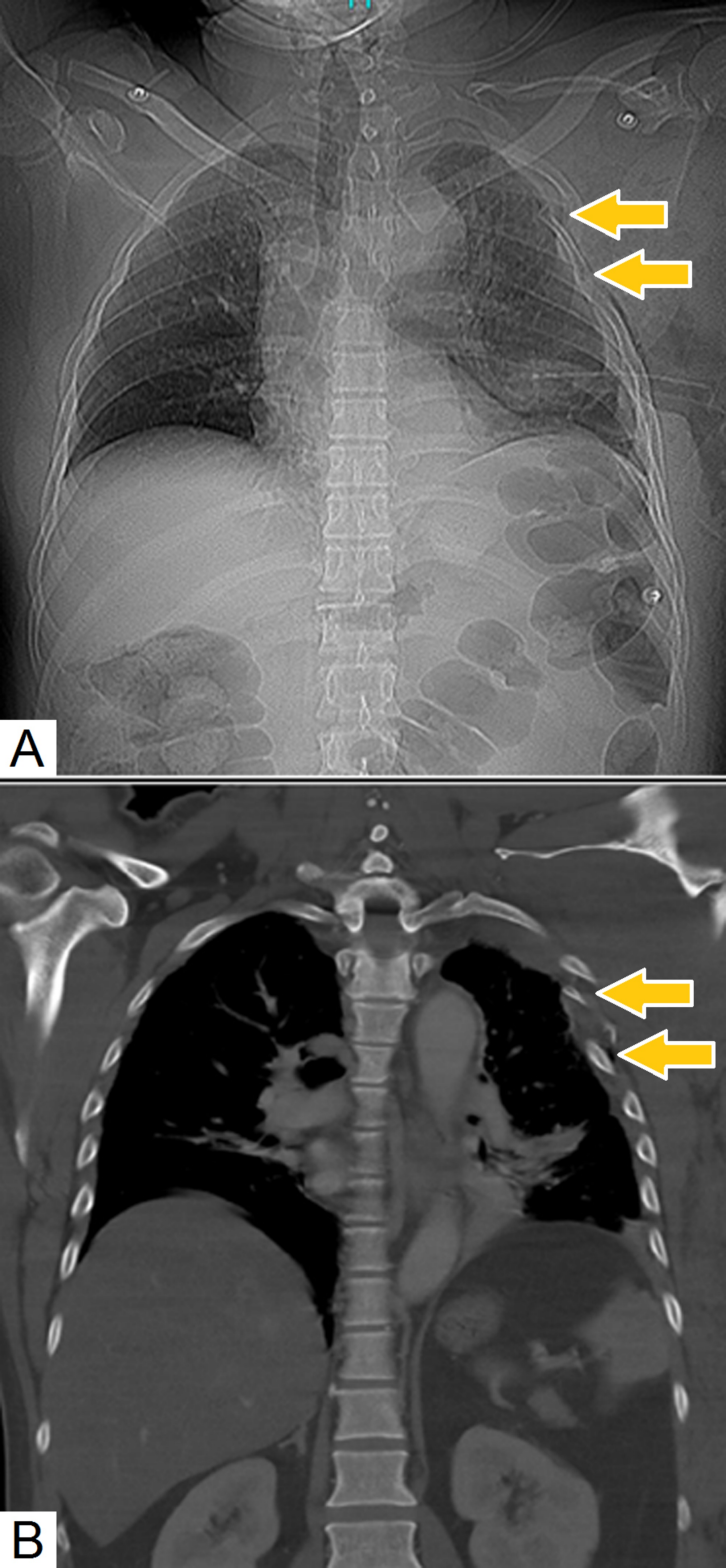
Comparison of scout film and thoracic computed tomography (A) Scout film demonstrating multiple left-sided rib fractures (arrows). (B) Coronal chest computed tomography (CT) images confirm the presence of multiple left-sided rib fractures (arrows).
